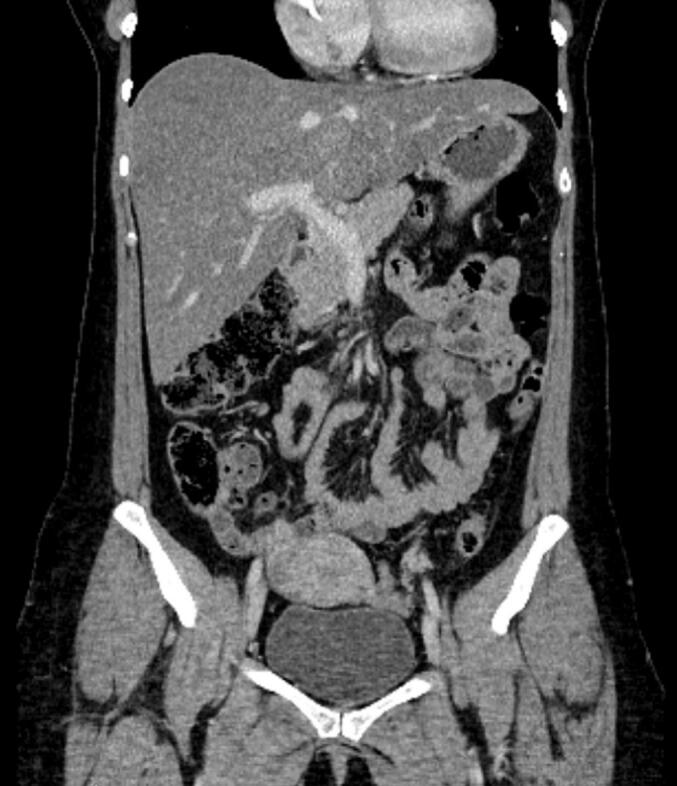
CT scan of the third patient 4 months after embolization of several branches of both uterine arteries.
